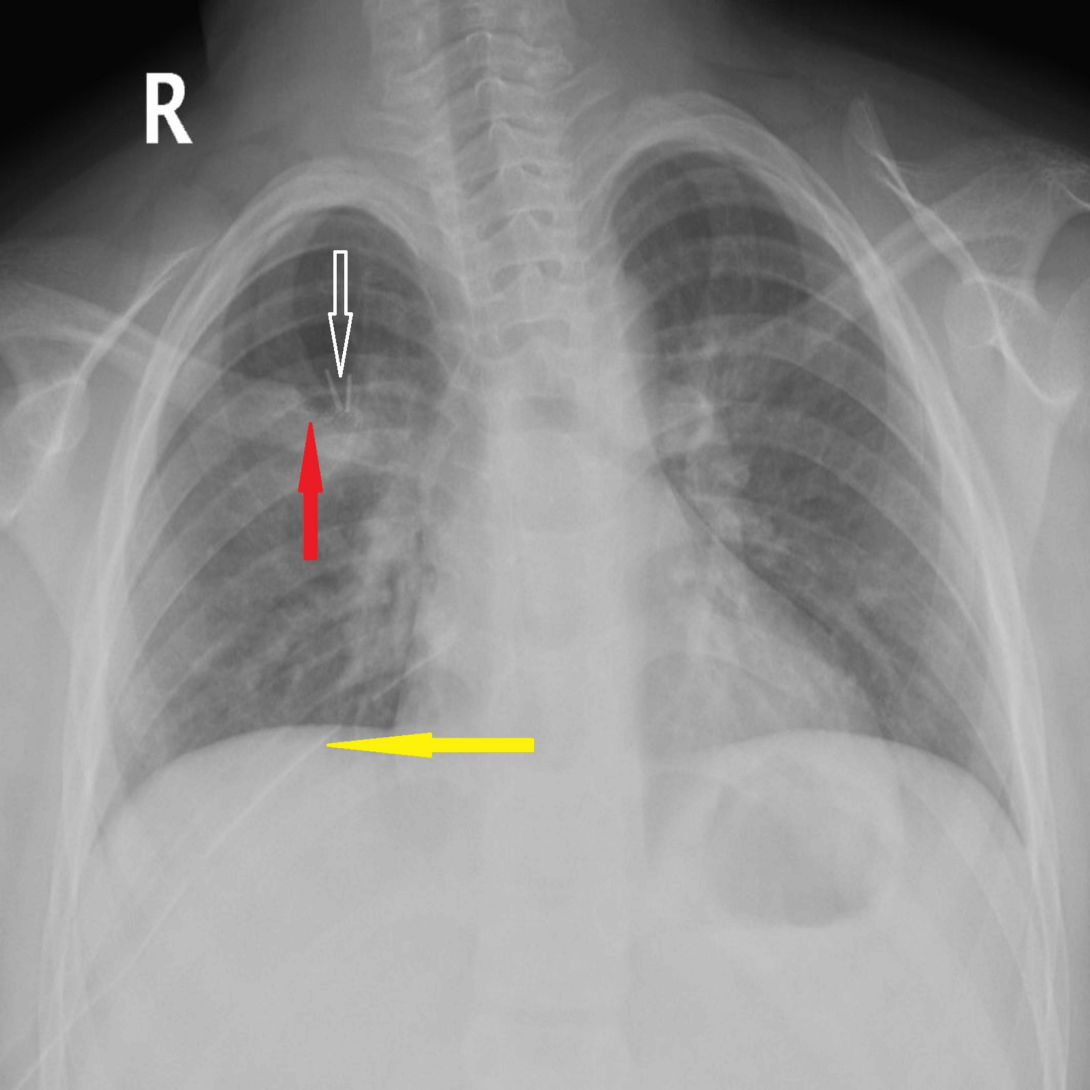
Postoperative chest radiograph demonstrating complete right lung re-expansion Postoperative chest X-ray showing complete re-expansion of the right lung following surgical intervention. Key features are indicated with arrows: Yellow arrow (left): Chest tube in situ Red arrow (up): Stapler line from bullectomy Wide hollow arrow: Endoscopic clips used during pleurodesis
